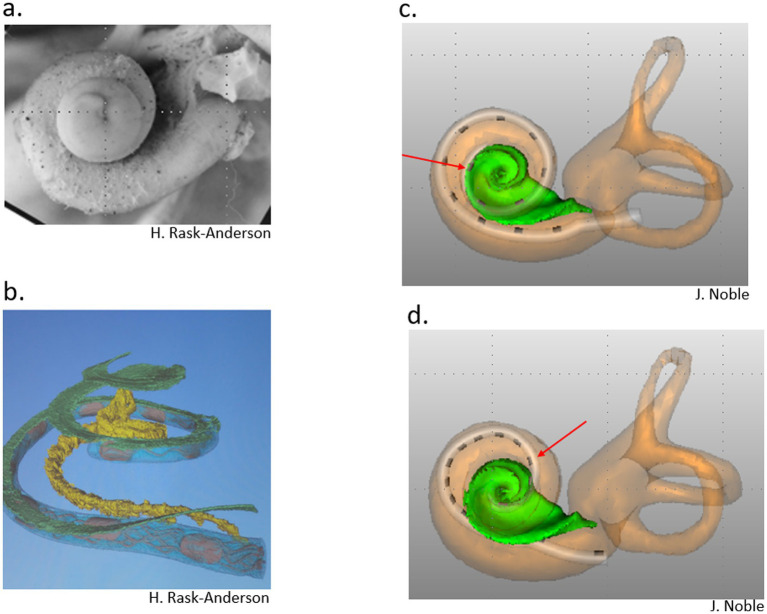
(a) Plastic mold of a human cochlea. (b) Synchrotron image of a cochlea with the bony matrix digitally removed showing the basilar membrane in green, the spiral ganglion in yellow and an electrode array in blue with individual electrodes in red. (c) Computer reconstruction from CT scan of a 28 mm electrode array in a patient. The most apical contact is at the 390 Hz SG frequency. (d) Computer reconstruction of 16.5 mm electrode array in a patient. The most apical contact is at the 629 Hz SG frequency. Red arrows point to the most apical electrode in each array. Spiral ganglion is colored green. CT and synchrotron images of longer and shorter electrode arrays in the cochlea.
